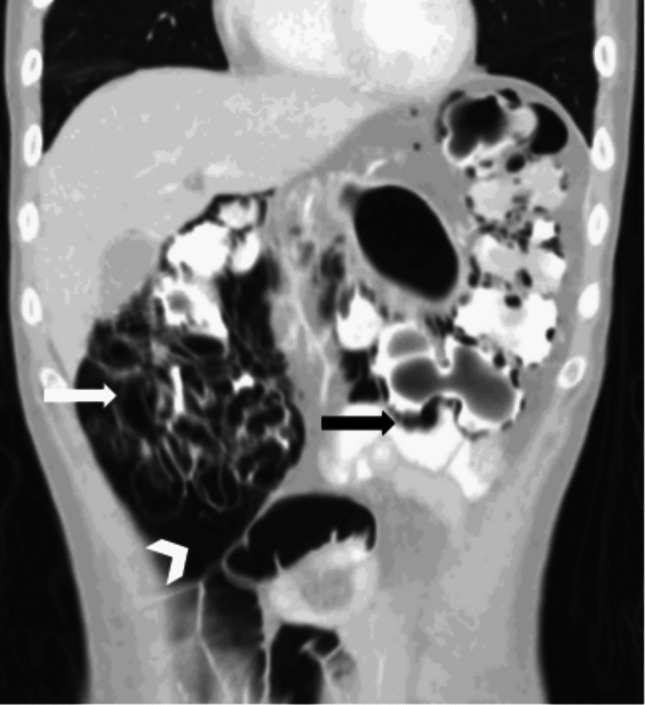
34-year-old male with HIV/AIDS on high dose steroids. Coronal CT image shows PI of the colon (arrows) and adjacent pneumoperitoneum (arrowhead). This patient was asymptomatic and treated with conservative management including bowel rest and intravenous antibiotics
